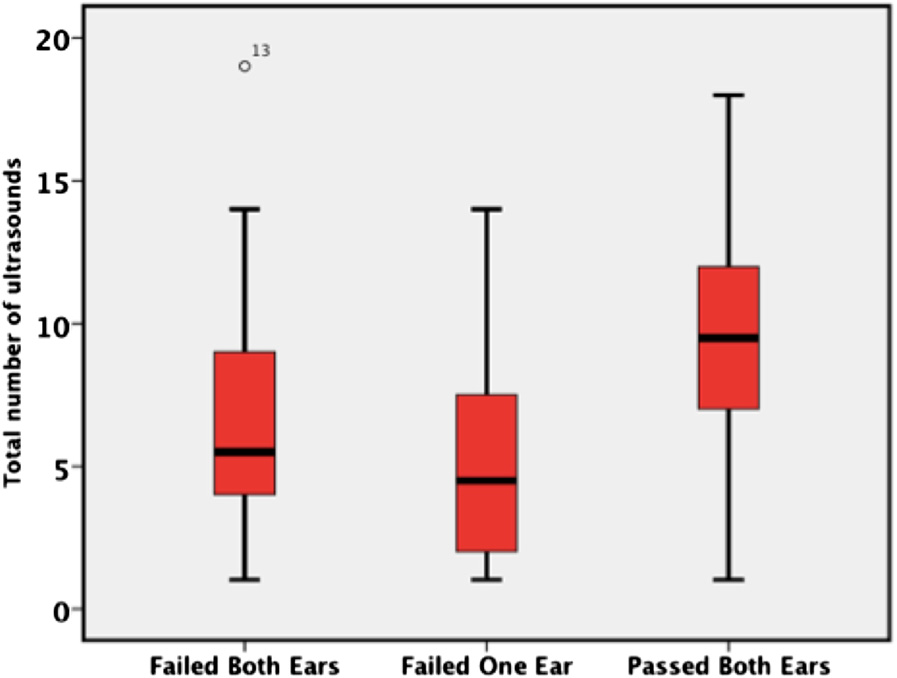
Hearing status and total ultrasound exposure.
